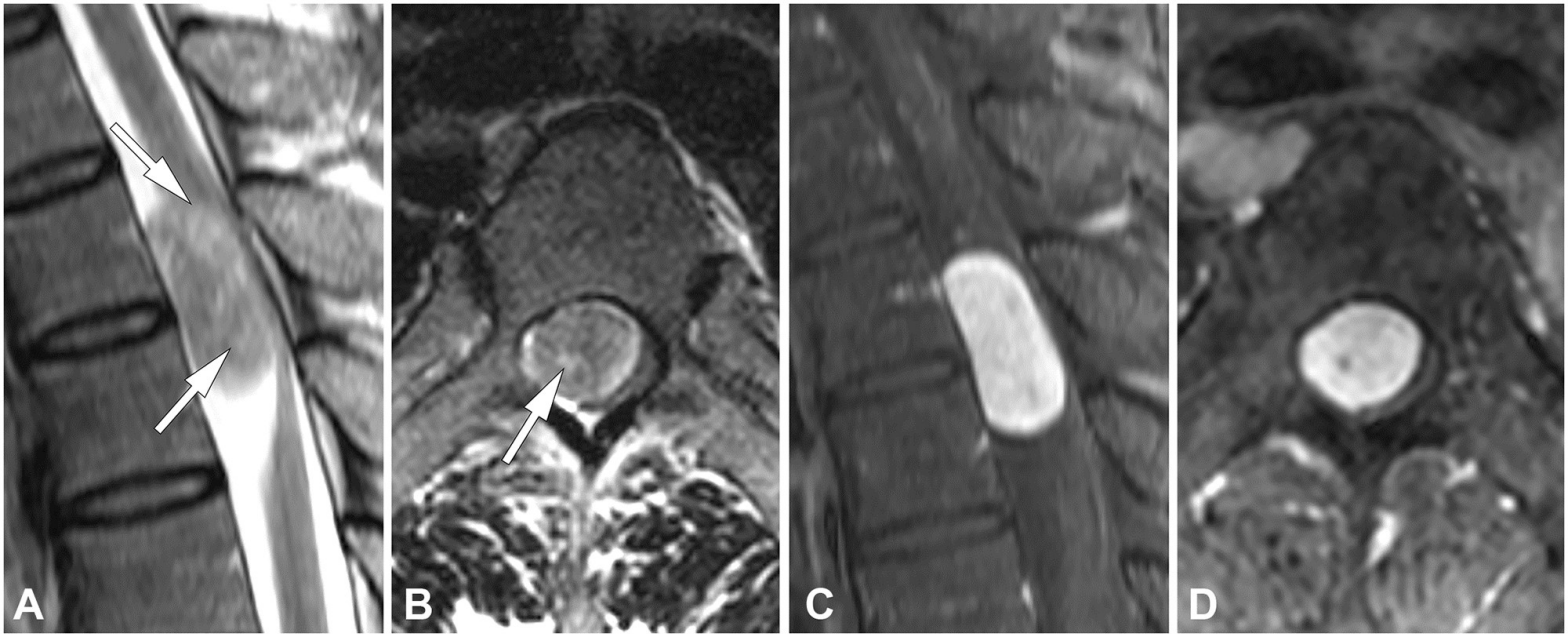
A schwannoma in a 42-year-old man. (A) Sagittal and (B) axial T2-weighted images show an intradural extramedullary mass on the T4-5 level with immeasurably small foci of fluid signal within the tumor (arrows), which was considered to represent cystic change. (C) Sagittal and (D) axial fat-suppressed contrast-enhanced T1-weighted images show relatively homogeneous enhancement.
